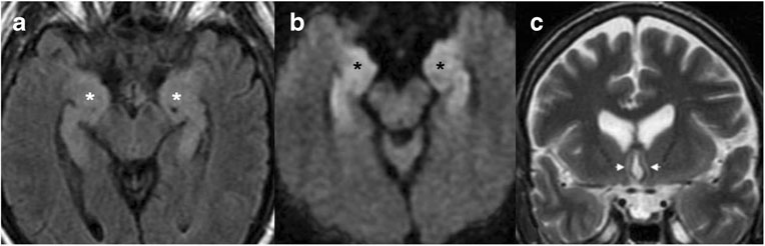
a–c A 66-year-old male with confusion 10 days after allogeneic bone marrow transplantation. Increased T2/FLAIR signal and restricted diffusivity are noted in the mesial temporal lobes (*asterisks* in a, b), and forniceal columns (*arrowheads* in c), compatible with HHV 6 encephalopathy
